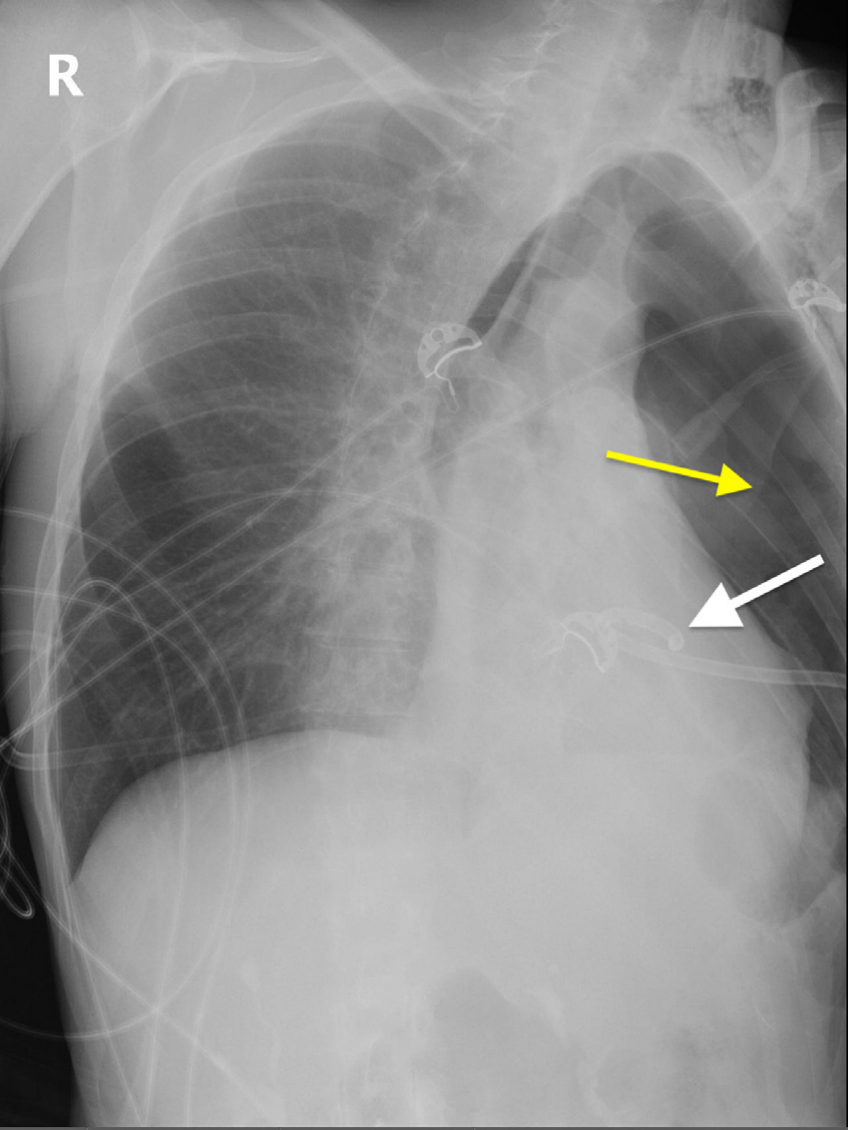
Portable 30-degrees upright chest radiograph showing interval placement of the chest tube with the tip projecting onto the cardiac silhouette (white arrow), along with the left sided pneumothorax (yellow arrow).
